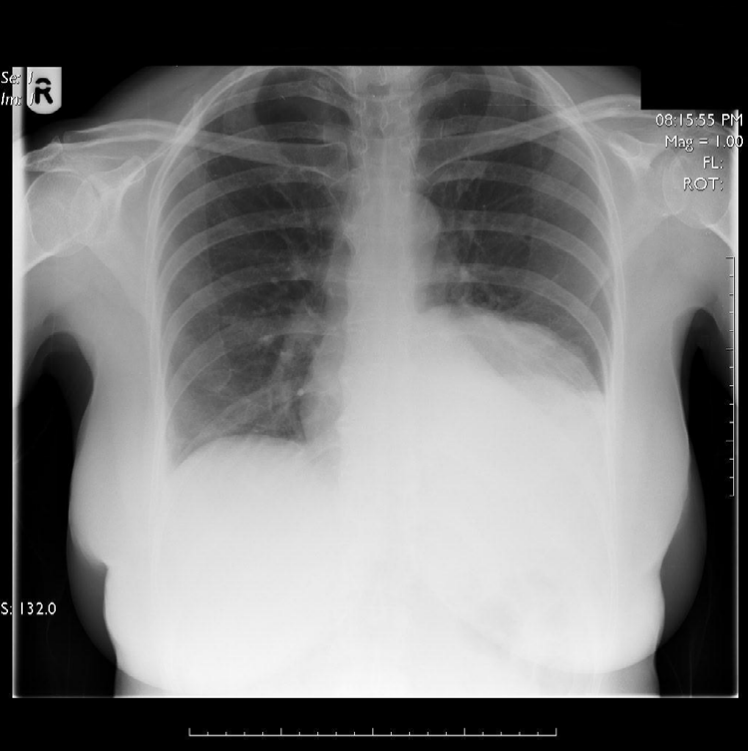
Chest radiograph showing a raised left hemi-diaphragm and volume loss in the left lower lobe.
